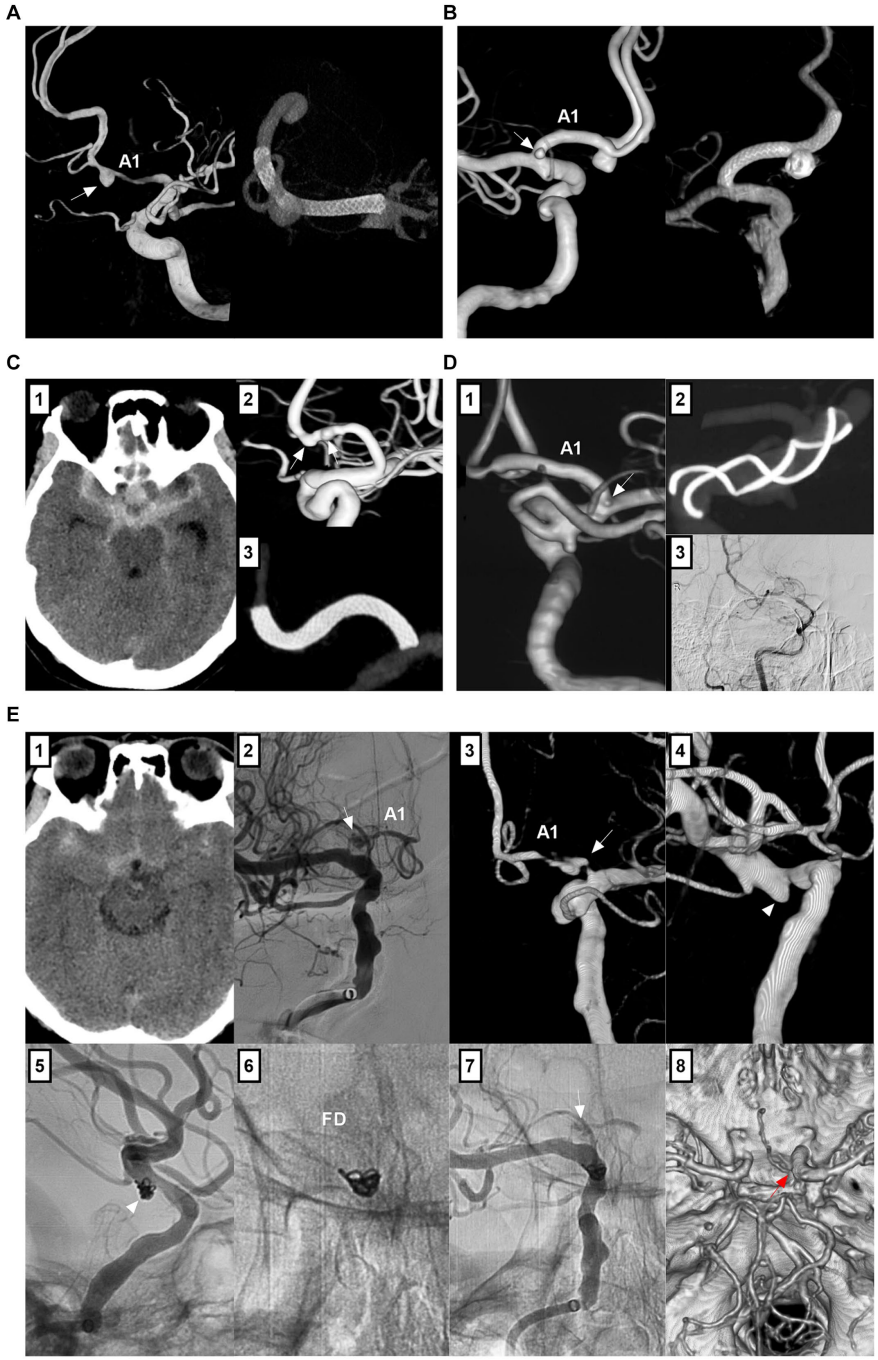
Treatment of A1 aneurysms by FD deployment. (A) Left panel: 3D-DSA showing an A1 dissecting aneurysm (arrow); Right panel: Vaso-reconstructive image showing the FD covering the aneurysm. (B) Left panel: 3D-DSA showing tandem A1 dissecting (arrow) and AcomA aneurysms; Right panel: Vaso-reconstructive image showing the FD covering these two aneurysms, the AcomA aneurysm was coiled. (C) Panel 1: CT showing subarachnoid hemorrhage; Panel 2: 3D-DSA showing two tandem A1 dissections (arrows); Panel 3: Vaso-reconstructive DSA showing the FD covering the two dissections. (D) Panel 1: 3D-DSA showing an aneurysm of posterior communicating artery and an A1 aneurysm (arrow) at its origin; Panel 2: Vaso-reconstructive DSA showing the FD deployment covered A1 origin; Panel 3: Six-month follow-up DSA showing the vessel stenosis in the FD. (E) Panel 1: CT showing subarachnoid hemorrhage; DSA (panel 2) and 3D-DSA (panel 3) showing the A1 origin dissecting aneurysm (arrows); Panel 4: 3D-DSA showing associated posterior communicating artery aneurysm (arrow); Panel 5: Unsubtracted DSA showing the coiled posterior communicating artery aneurysm (arrow); Panel 6: X-ray film showing that the FD covered the A1 origin; Panel 7: Unsubtracted DSA showing the decreased blood flow filled in the A1 dissection (arrow); Panel 8: Postoperative next day CTA showing that the A1 dissecting aneurysm (arrow) cannot be seen clearly. 3D, three-dimensional; A1, first segment; ACA, anterior cerebral artery; AcomA, anterior communicating artery; CT, computed tomography; CTA, computed tomography angiography; DSA, digital subtracted angiography; FD, flow diverter; ICA, internal carotid artery.
